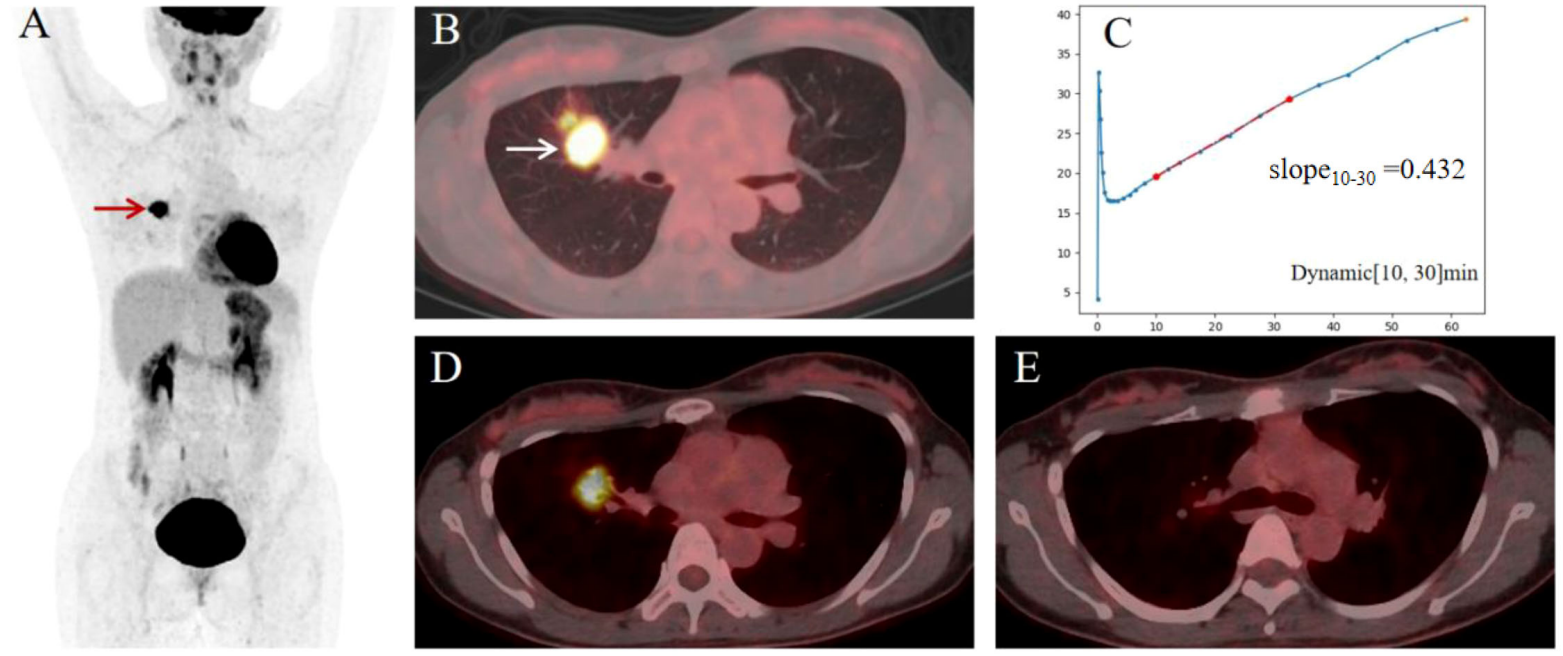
A 35-year-old female patient with newly diagnosed lung adenocarcinoma underwent dynamic and static PET/CT imaging for pre-treatment staging. (A) The maximum intensity projection image (MIP) of static ^18^F-FDG PET/CT. (B) Static PET/CT showed an FDG-avid solid nodules (2.6 × 2.0cm) in the upper lobe of the right lung (white arrow, SUVmax of 15.2, MTV of 4.7, TLG of 44.2). (D, E) showed no suspicious metastatic lymph nodes. (C) Visualization of the feature Slope_10–30_ within the TAC of dynamic PET. Right upper lobectomy and systematic lymph node dissection were performed within 1 week after PET/CT examination. Further surgical pathology identified lung adenocarcinoma with LNM, pT3N1.
